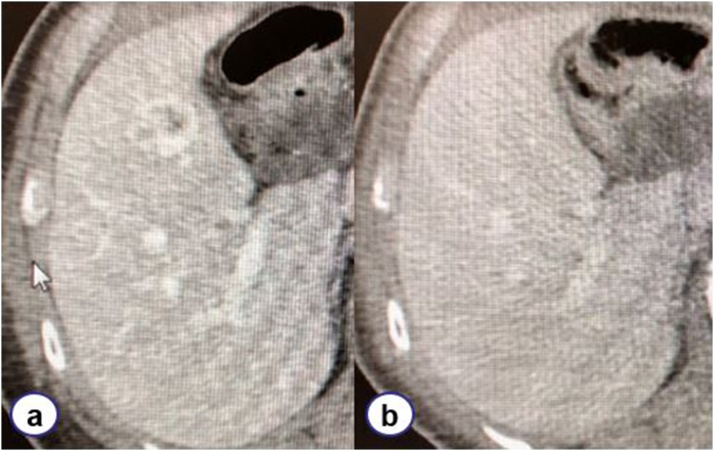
Computed tomography imaging. On computed tomography imaging conducted 2 years postoperatively, a mass with ring enhancement measuring 22 × 15 mm in S5 emerged (a) compared to the scan performed 1 year postoperatively (b).
